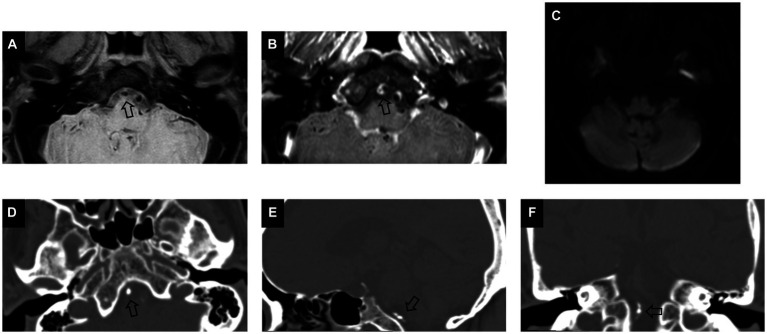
Example of calcification presence in a non-culprit plaque of a 66-year-old female asymptomatic patient. Pre-contrast (A) and post-contrast (B) vessel wall MRI images show an eccentric plaque (arrow) in V4 segment of the vertebral artery. DWI image (C) demonstrates no presence of hyperintensity in the posterior circulation. Axial (D), sagittal (E), and coronal (F) CT images demonstrate the corresponding presence of calcification (arrow), which is classified as multiple, non-spotty, and non-intimal predominant calcification on visual assessment.
